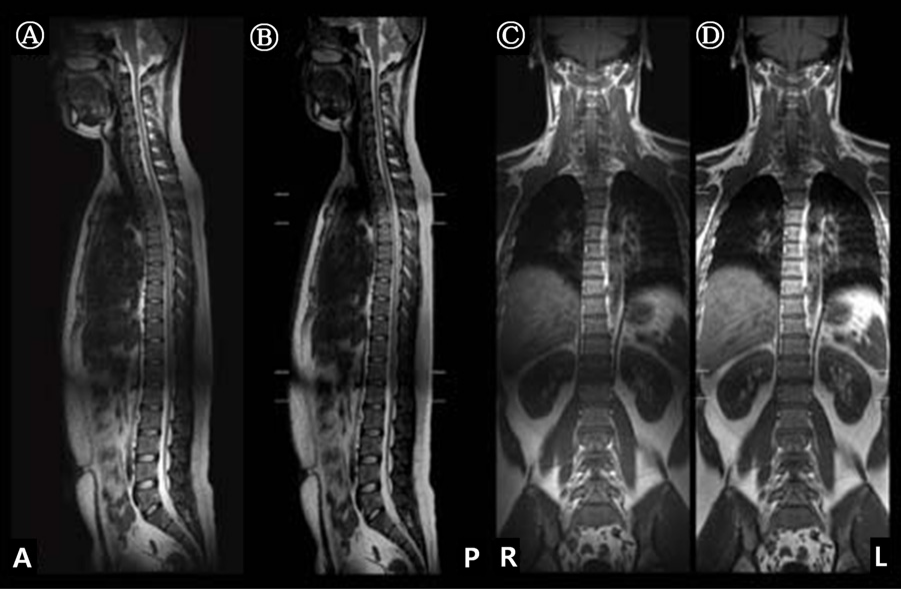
A representative subject’s stitching results for the use of SIFT algorithm on GUI configuration (A; sagittal section and C; coronal section) and the use of stitching algorithm commercially mounted on the MRI system (B; sagittal section and D; coronal section). All images acquired from 3.0 T MRI system.
